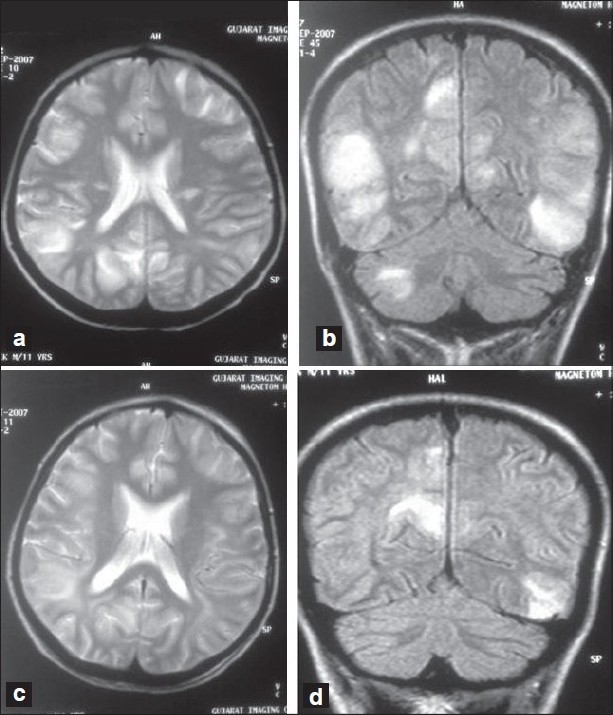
T2 weighted (a) and FLAIR (Fluid attenuated inversion recovery) MRI images (b) showing patchy areas of confluent hyperintensities involving cortical and subcortical, bilateral, frontal, posterior temporal, parietooccipital lobes and right cerebellum. MR, one week after steroid treatment, showing resolution of lesions in T2 weighted (c) and FLAIR- (d) images
